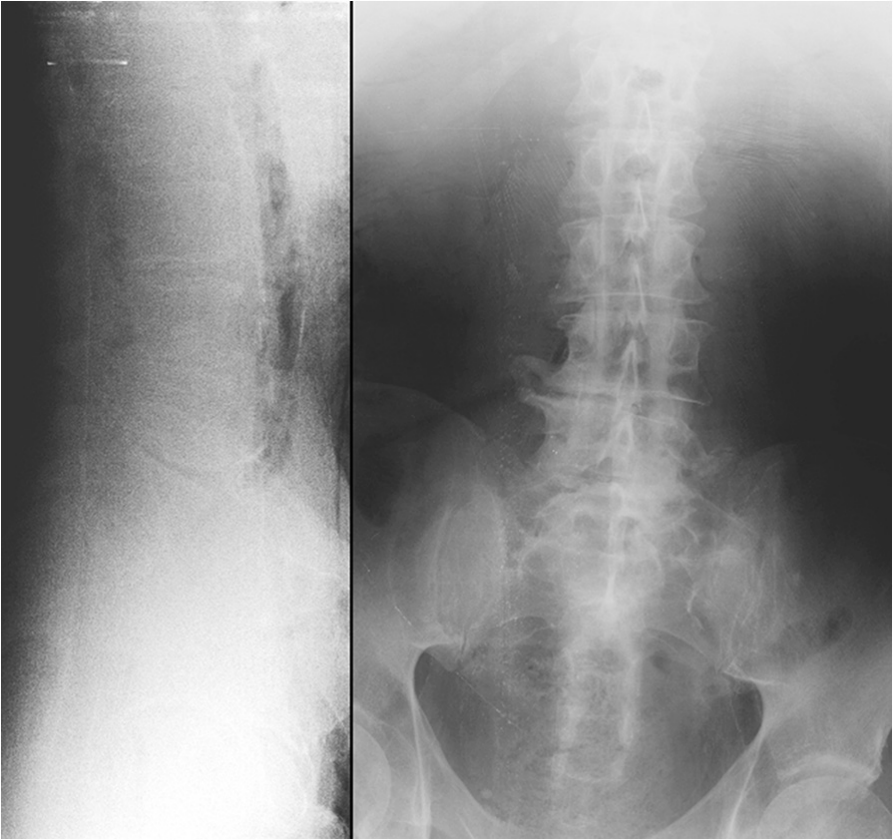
Poor quality of lateral and AP x-ray of the lumbar spine and pelvis. Due to the sagging of abdominal organs and additional gas artefacts in the abdominal aorta in lateral views scarcely any structures were identifiable, in AP views the lumbar spine and pelvic bones were poorly detectable.
